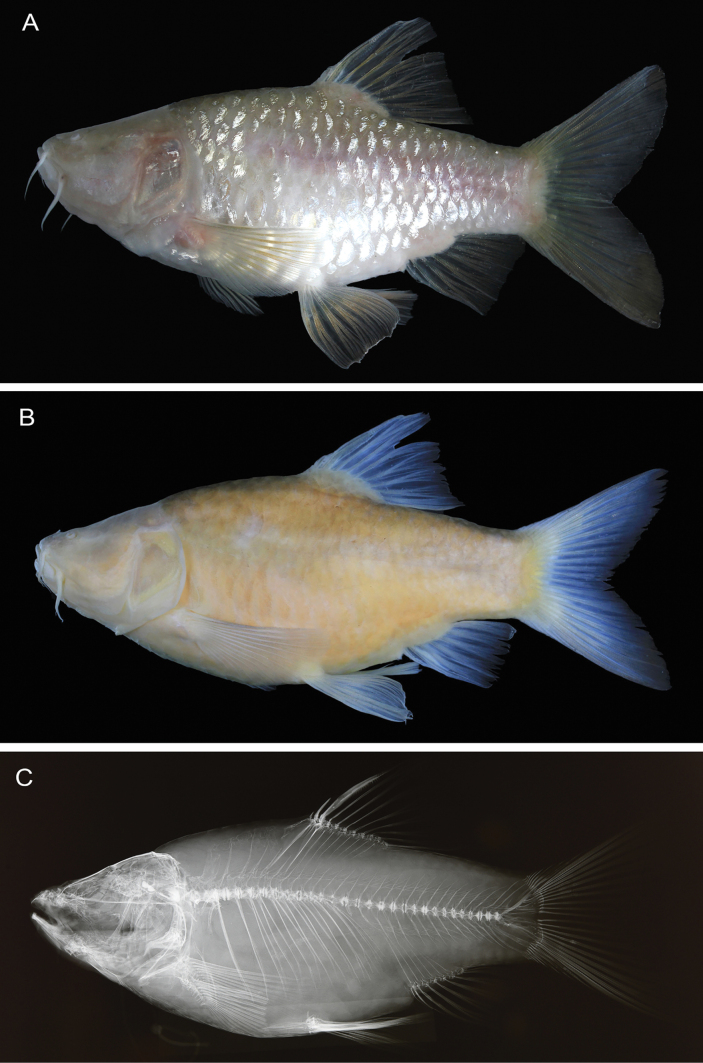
Barbodesklapanunggalensis* sp. nov., MZB.26656, paratype, 73.0 mm SL A fresh B preserved C radiograph.
